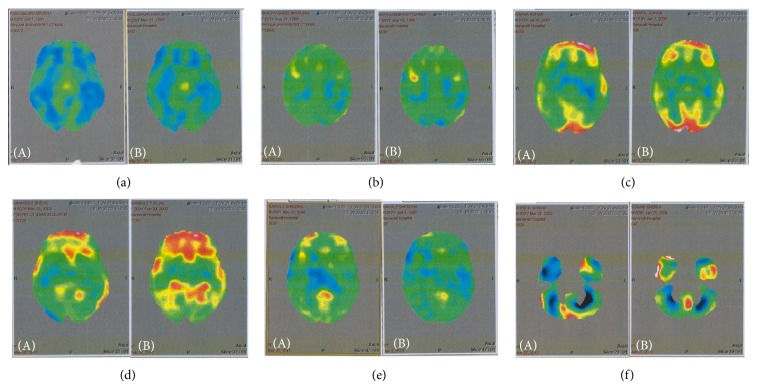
Improvements in PET-CT scan brain: PET-CT scan images of (A) pre- and (B) postintervention showing increased metabolic activity in various areas. Blue areas indicate hypometabolism, green areas indicate normal metabolism, yellow areas indicate slightly high metabolism, and red areas indicate high metabolism.
